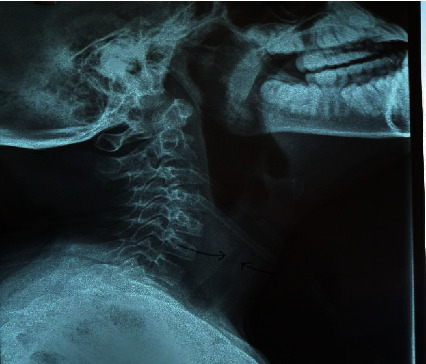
Lateral cervical X-ray showed partial narrowing in the region of the cricoid cartilage (arrowed).
